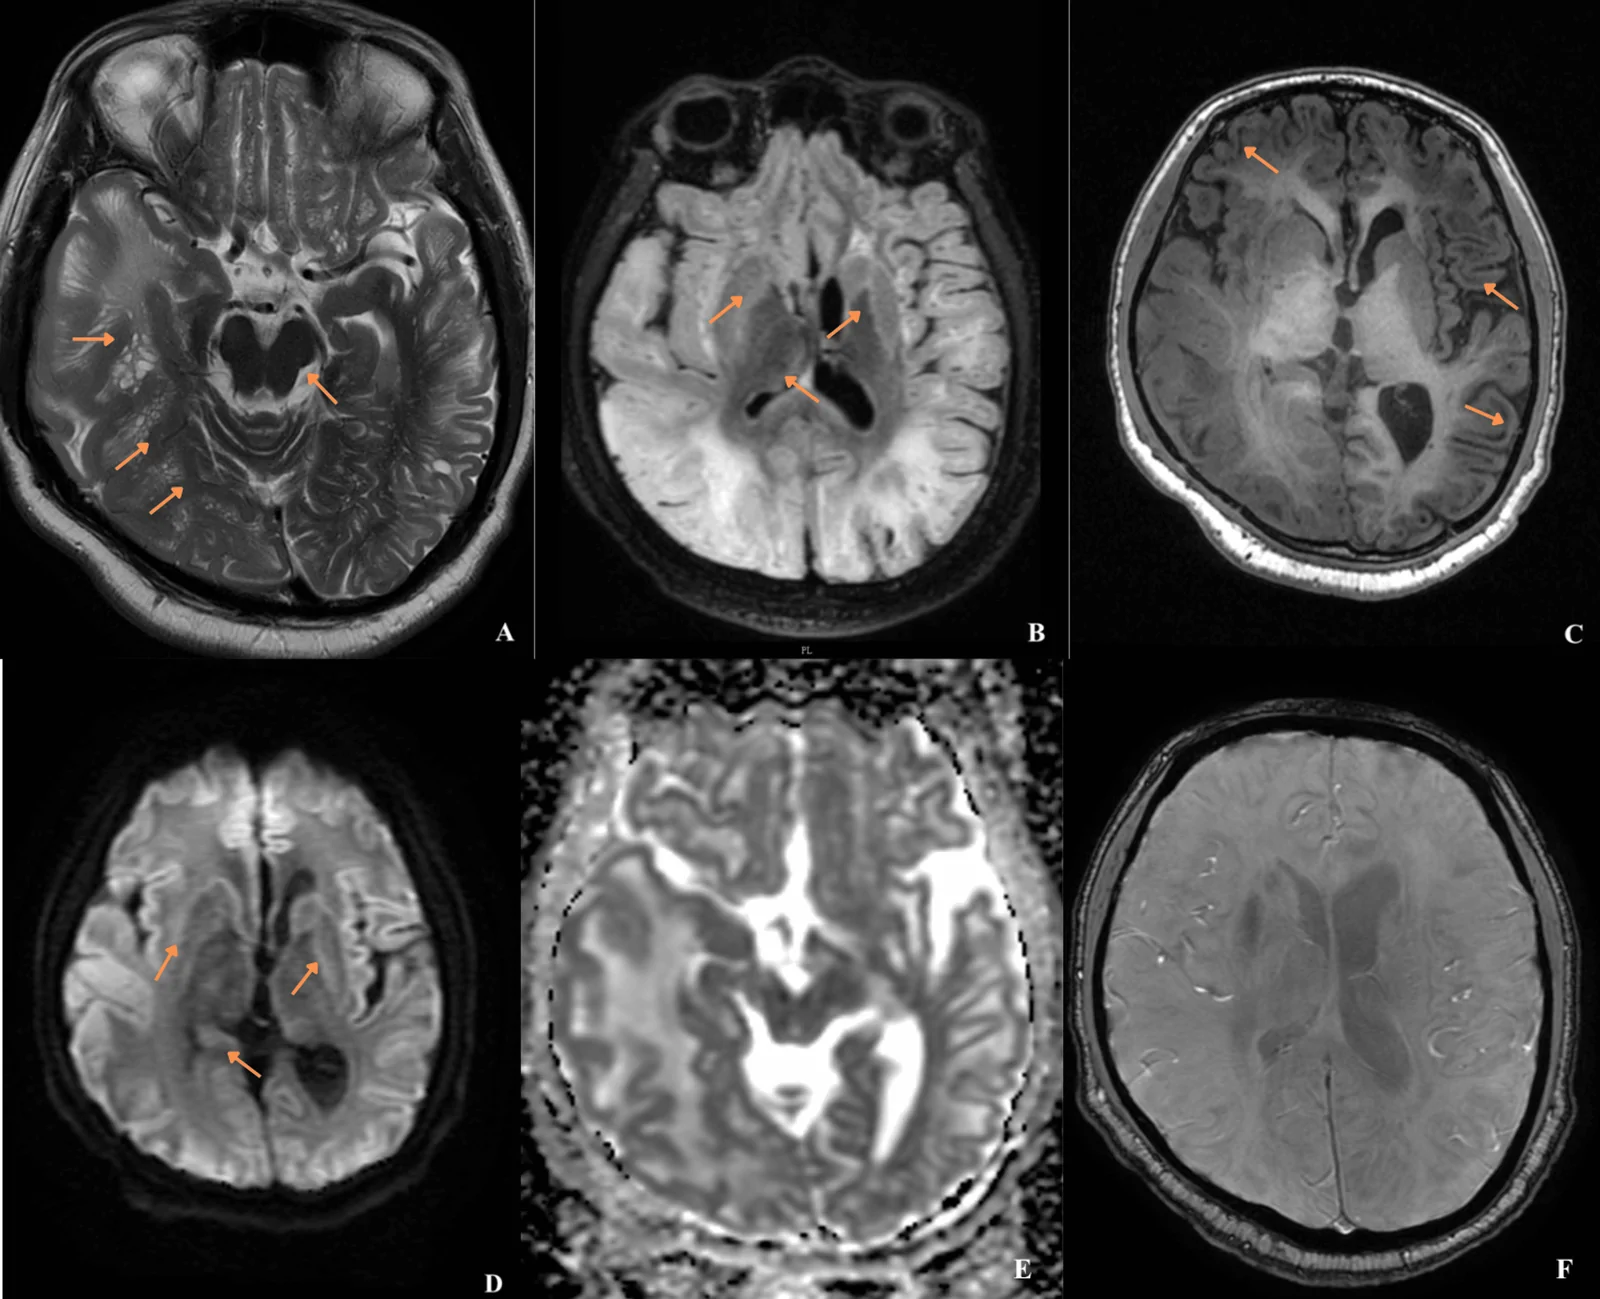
MRI findings (A) T2-weighted images show diffuse involvement of the deep and subcortical white matter along with cystic changes and prominent perivascular spaces. The brainstem is characteristically spared. (B) FLAIR imaging shows the characteristic centripetal pattern of involvement with involvement of the deep and subcortical white matter and bilateral basal ganglia and right thalamus. Periventricular white matter and corpus callosum are spared. (C) T1-weighted image shows diffusely hypointense white matter with U-fibre involvement. Periventricular white matter is spared. (D) DWI is consistent with FLAIR imaging, with no focus of diffusion restriction (E) or blooming (F) MRI: magnetic resonance imaging; FLAIR: fluid-attenuated inversion recovery; DWI: diffusion-weighted imaging
